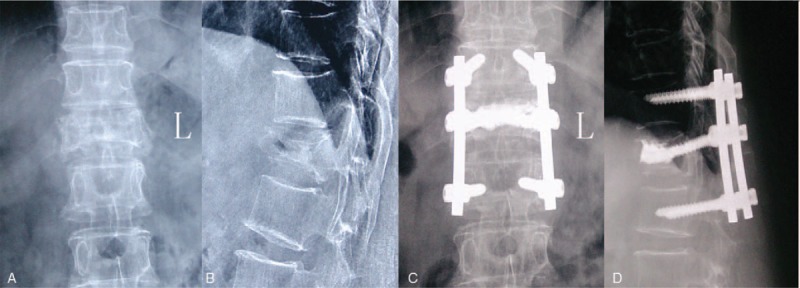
Representative case of posterior spinal fixation with vertebroplasty. Preoperative posteroanterior (A) and lateral (B) radiographs of a 69-year-old-female who underwent PSF+VP surgery for KD at T12. The preoperative local vertebral Cobb angle was 19°, which was corrected to 10° after surgery. Postoperative posteroanterior (C) and lateral (D) radiographs taken 2 years after surgery. Loss of correction was 1°.
